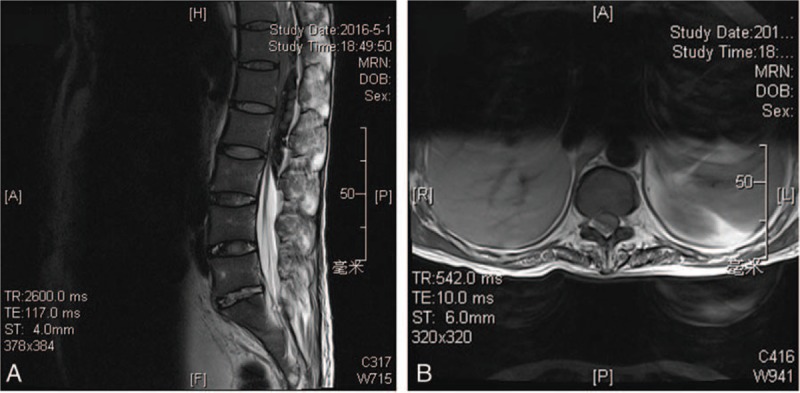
(A, B) MRI showed epidural hematoma was formed from T11 to L3 level and compression of the dural sac. (A) The sagittal image; (B) the cross-sectional image. MRI = magnetic resonance imaging.
